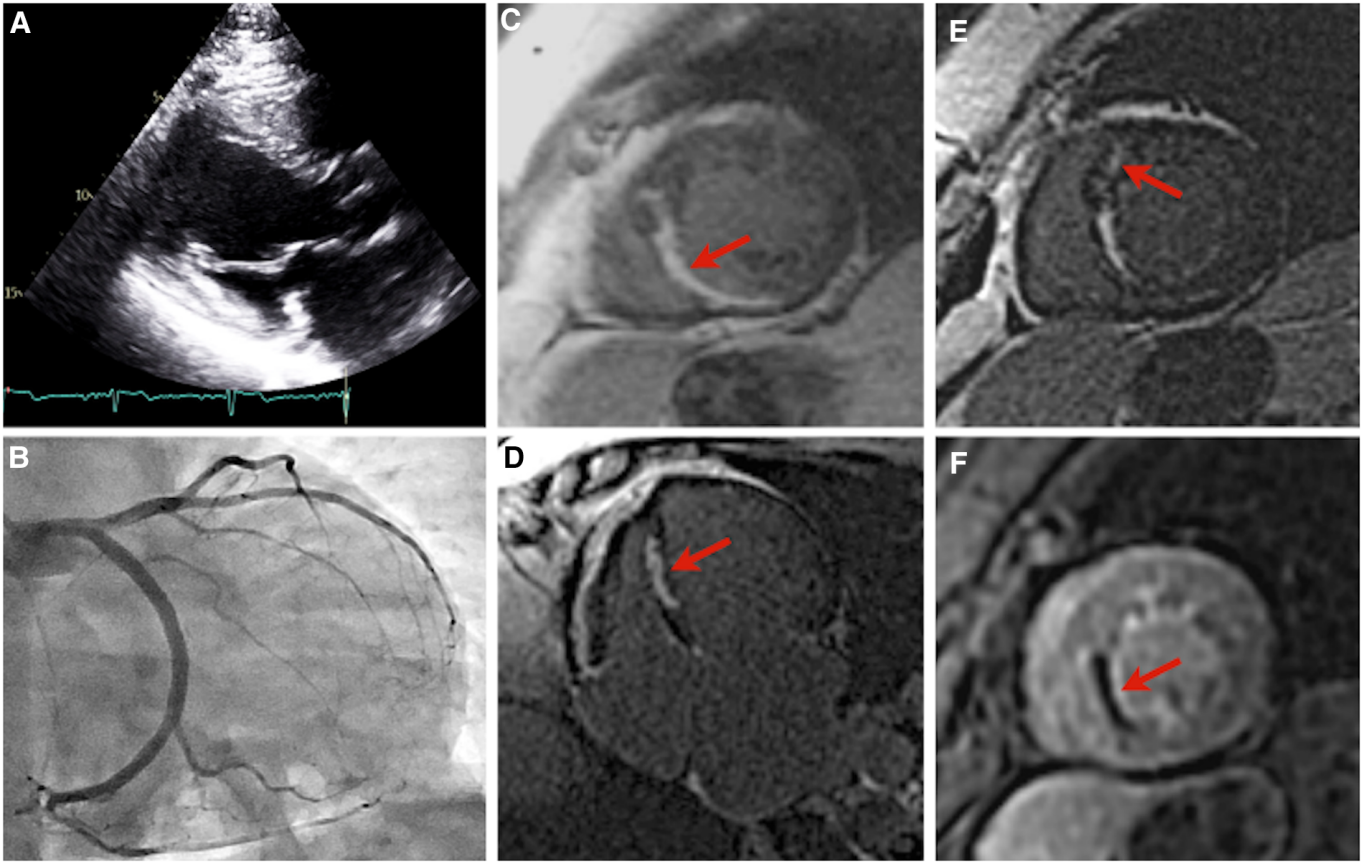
Imaging of patient B. Parasternal long axis echocardiogram, with an interventricular septal thickness measurement of 11 mm during diastole, and a patent left ventricular outflow tract (A). Right anterior oblique caudal angiogram demonstrating a diminutive left anterior descending artery (B). Images (C–F): cardiac magnetic resonance imaging of patient B. Near-transmural delayed gadolinium enhancement of the mid inferior and inferoseptal wall segments, short-axis view (C). Near-transmural delayed gadolinium enhancement of the mid inferior and inferoseptal wall segments, four-chamber view (D). Focus of mid-myocardial delayed gadolinium enhancement in the mid anteroseptal wall segment, short-axis view (E). Near-transmural septal hypoperfusion, short-axis view (F).
